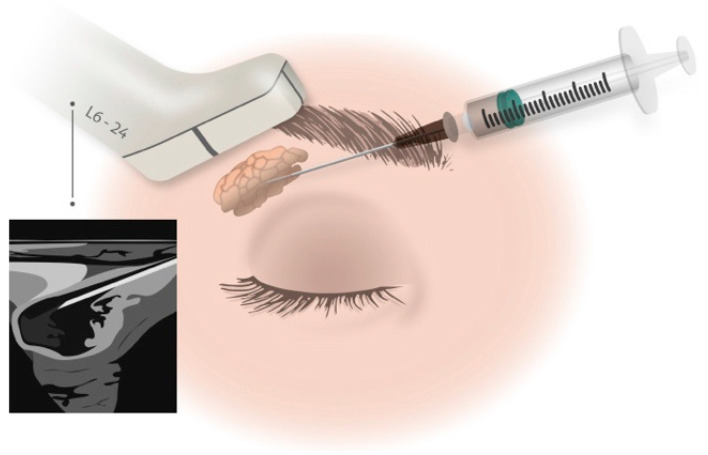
Administering an injection into the lacrimal gland with the assistance of ultrasonic guidance. (Reprinted without modifications from Moller-Hansen et al. [21]).
